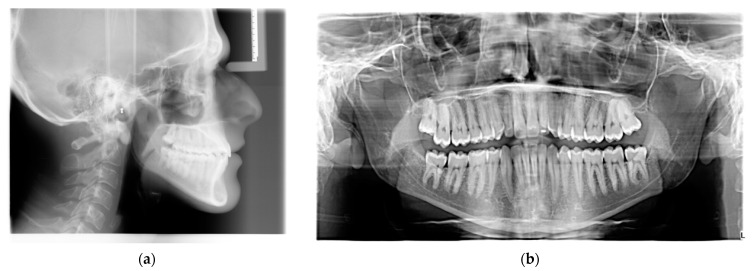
(a) Lateral cephalogram and (b) panoramic radiograph at the end of the treatment.
